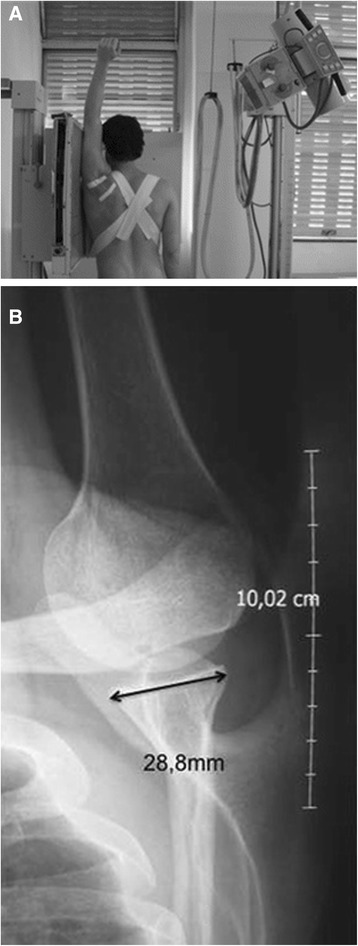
Bernageau Radiography. a) Patient positioning. b) Antero-posterior glenoid width measurement on this view (Murachovsky *et al.* [43])
